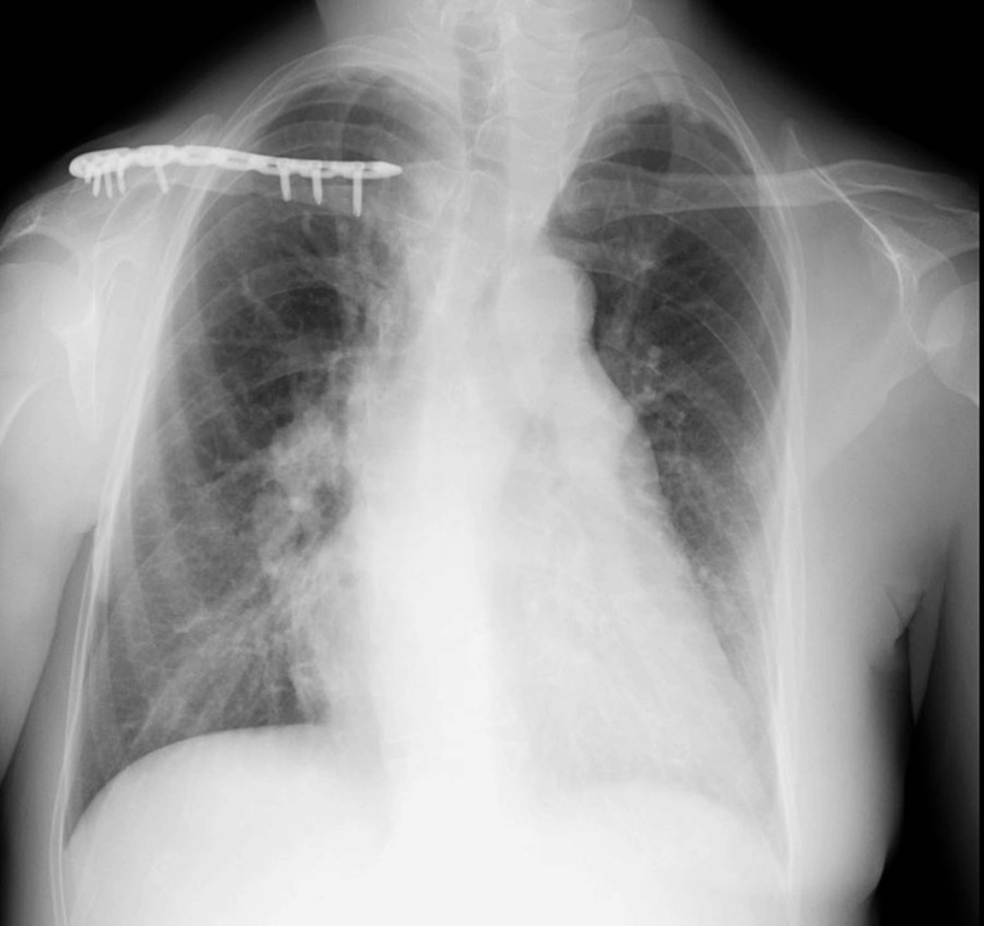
The patient's chest radiograph obtained on admission
